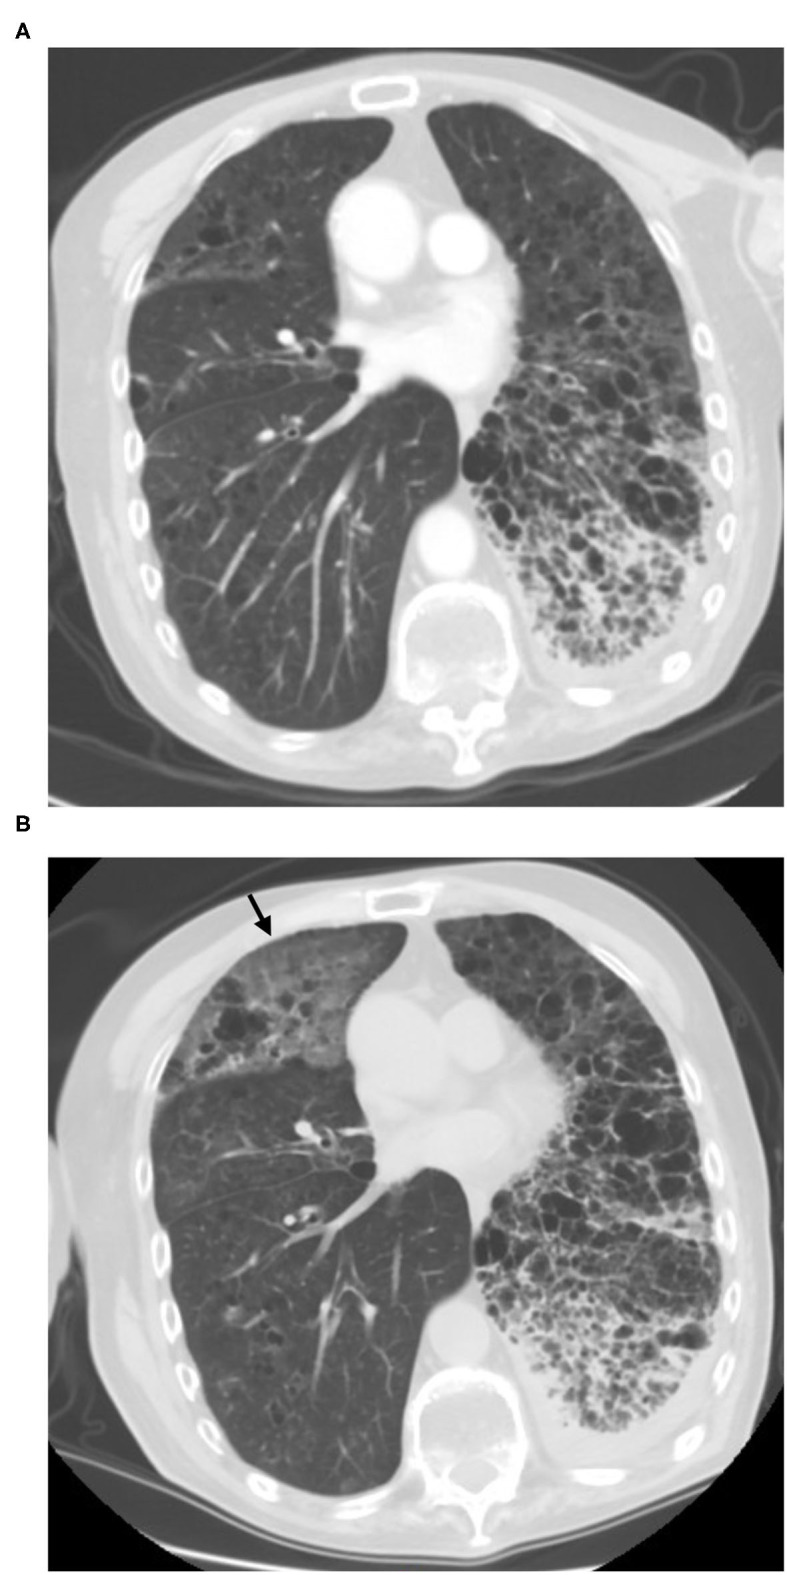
Sixty-six-years-old male with post-target therapy of lung adenocarcinoma history. Comparison with the transverse CT scan (A), the screening CT (B) shows new bilateral, peripheral GGO associated with smooth interlobular and intralobular septal thickening (crazy-paving pattern) (arrow).
